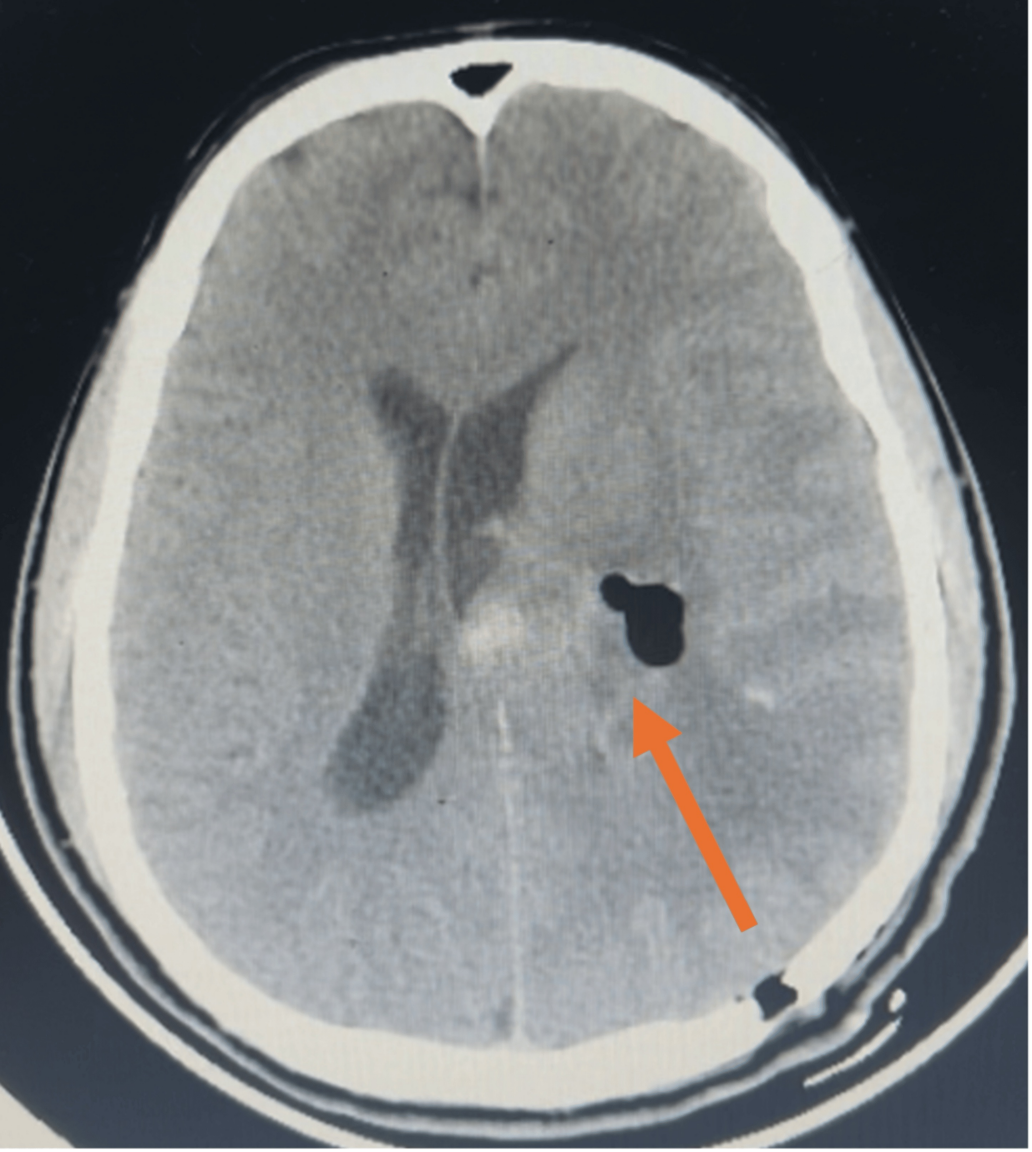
Postoperative non-contrast CT brain Postoperative CT brain plain highlighting the trajectory of the stereotactic biopsy
